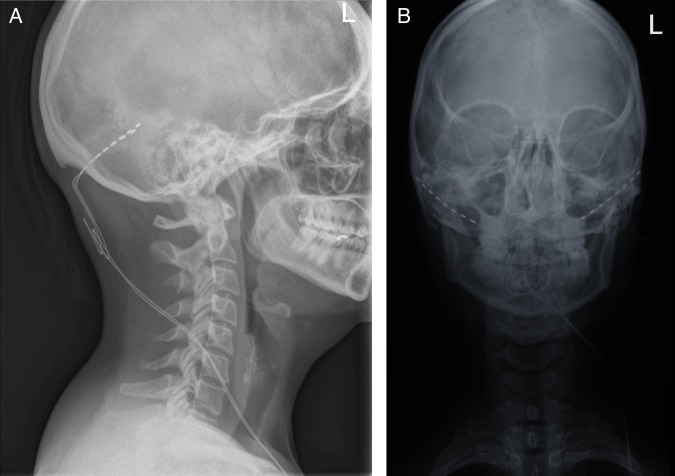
Skull X-rays showing occipital nerve stimulation electrode placement. Lateral (A) and anterior posterior (B) skull views showing the occipital nerve stimulation electrodes (dotted lines) and leads (solid lines).
